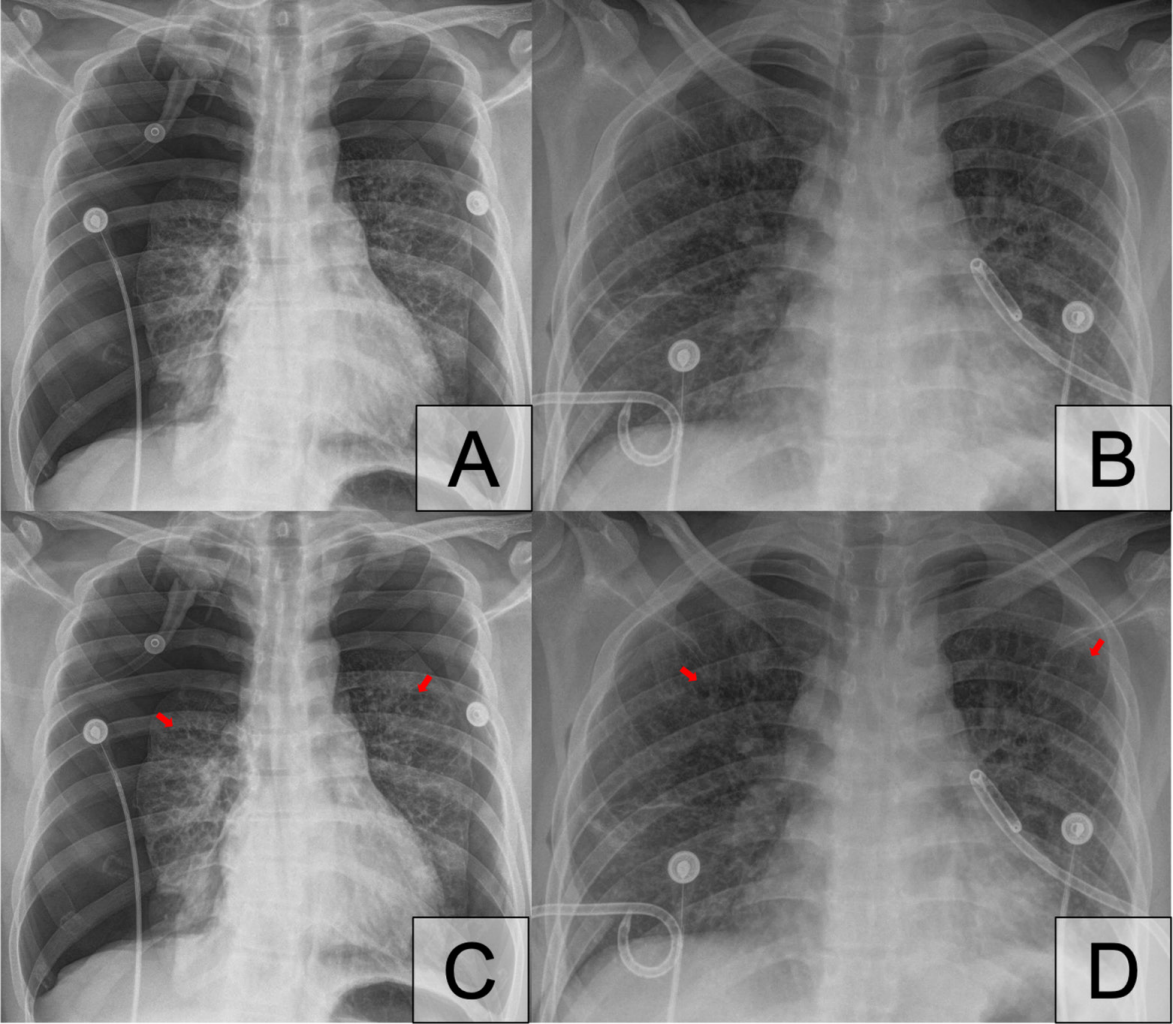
A: Chest radiograph on arrival to Emergency Department. B: Chest radiograph after insertion of bilateral chest tubes. C and D: Arrows indicating airspace lucencies.
